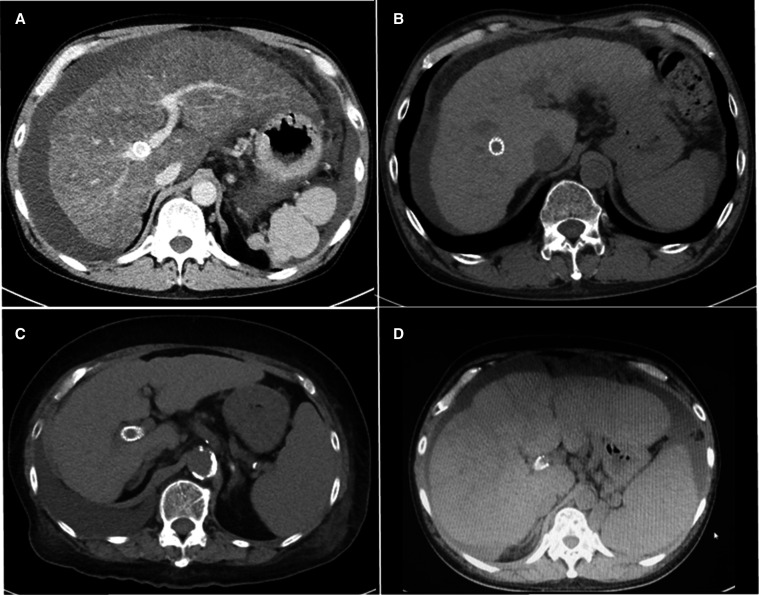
Abdominal computed tomography scan images post TIPS creation. (A) Case 1, (B) Case 2, (C) case 3 and (D) case 4. TIPS, transjugular intrahepatic portosystemic shunt.
